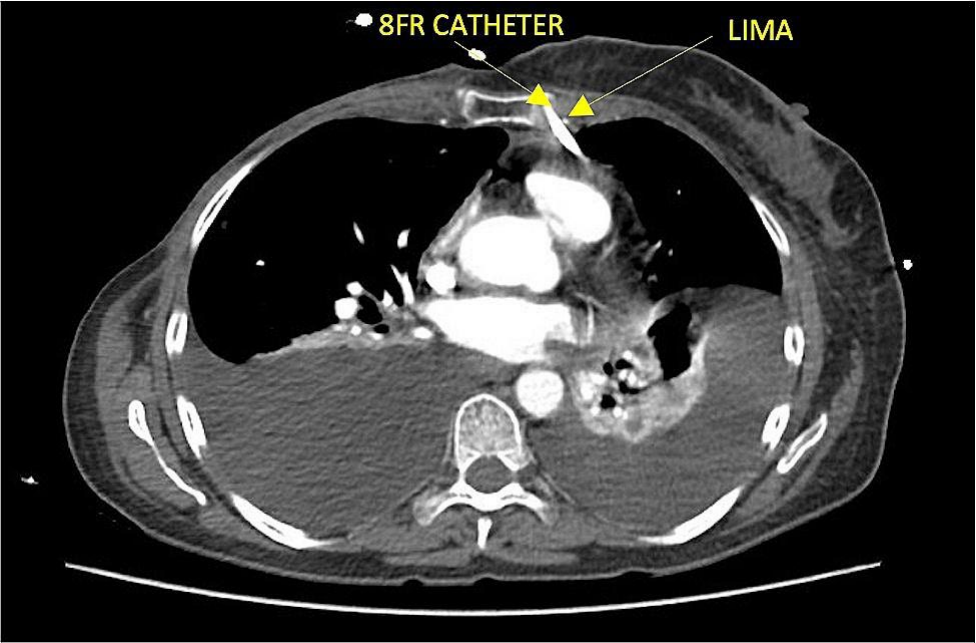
CT scan showing distance between the catheter passage and the LIMA. CT: computed tomography, LIMA: left internal mammary artery
